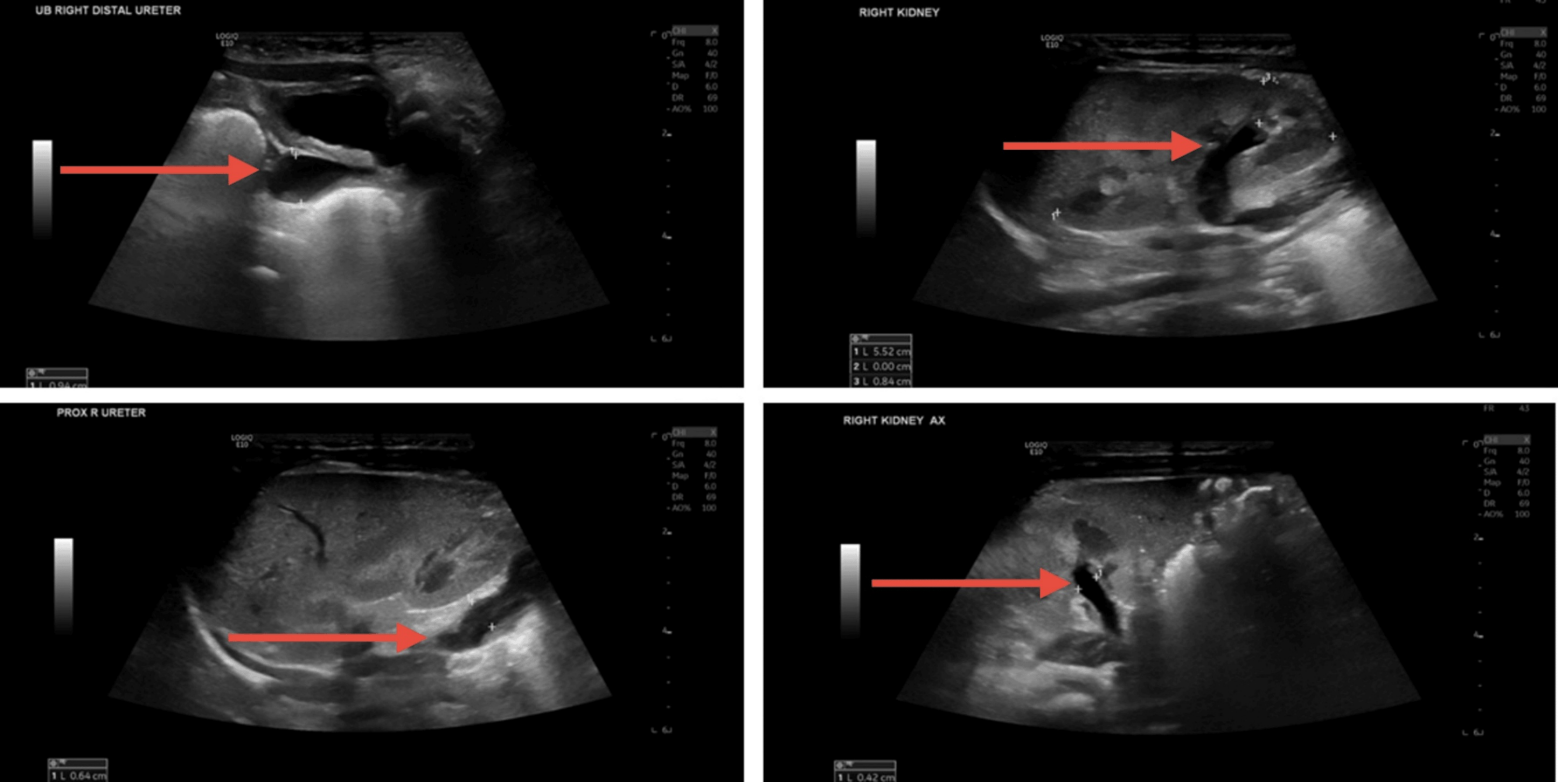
Ultrasound images Images demonstrate right-sided hydronephrosis and hydroureter, suggestive of obstructive uropathy. A dilated renal pelvis and right ureter, extending from the proximal to distal segments, were noted.
